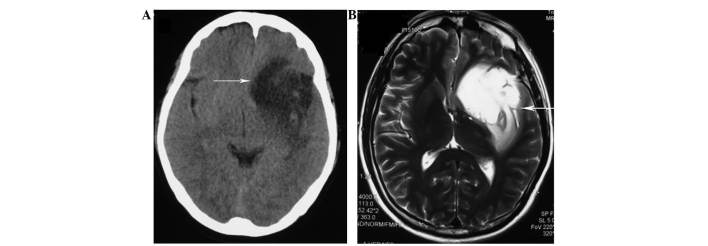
Cranial (A) computed tomography (CT) and (B) magnetic resonance imaging (MRI) showing an altered signal intensity in the left frontotemporal region of the brain (white arrow).
